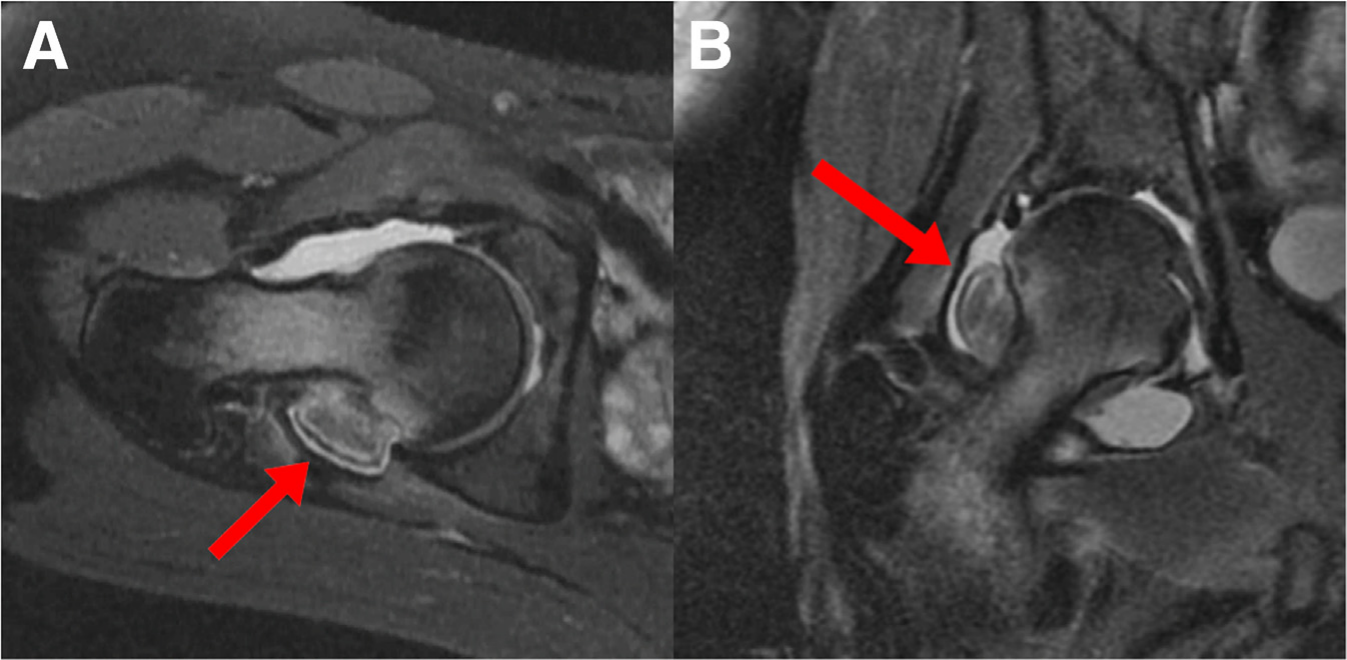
Preoperative T2-weighted magnetic resonance imaging of the right hip is shown. (A) Axial images of the right hip show the intra-articular mass (red arrow) at the posterior aspect of the femoral head-neck junction. (B) Coronal images of the right hip also show the position of the intraarticular mass (red arrow) along the posterior and lateral aspect of the femoral head–neck junction.
